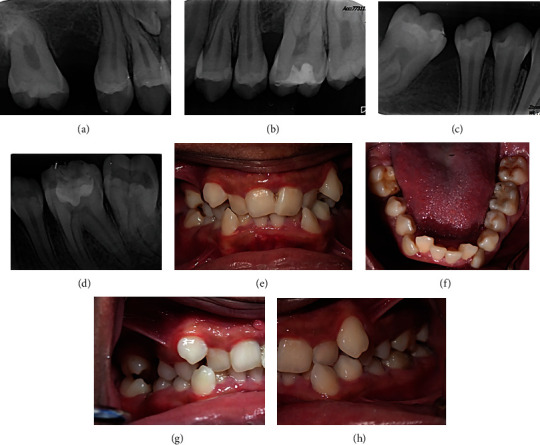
Periapical radiographs and intra-oral images taken at 6 months recall from second excisional biopsy (no recurrence was observed up to 12 months).
